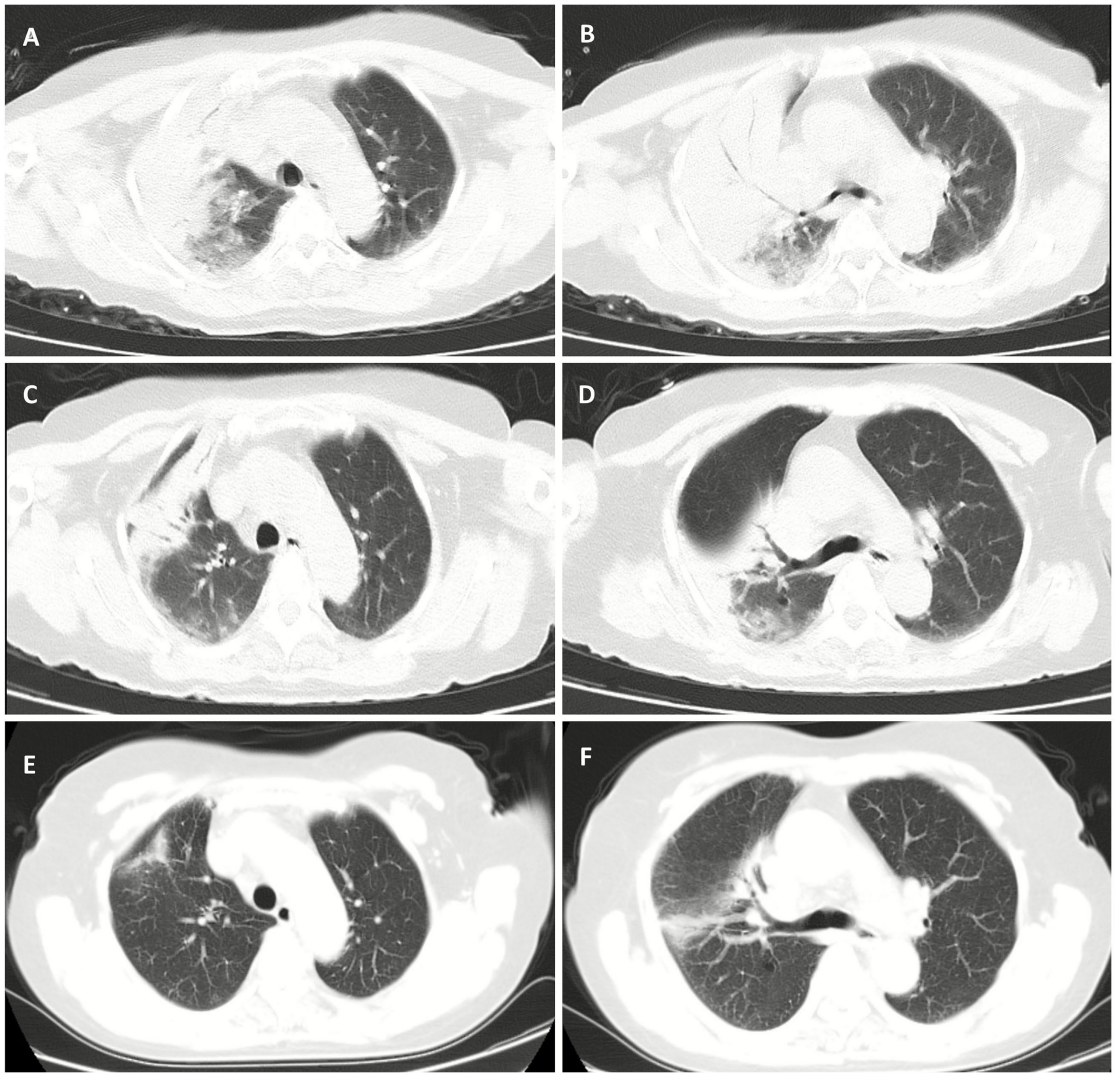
Serial chest CT images of the patient during hospitalization and follow-up. (A,B) At admission (day 1). (C,D) Day 15 of hospitalization. (E,F) 9 days after discharge.
